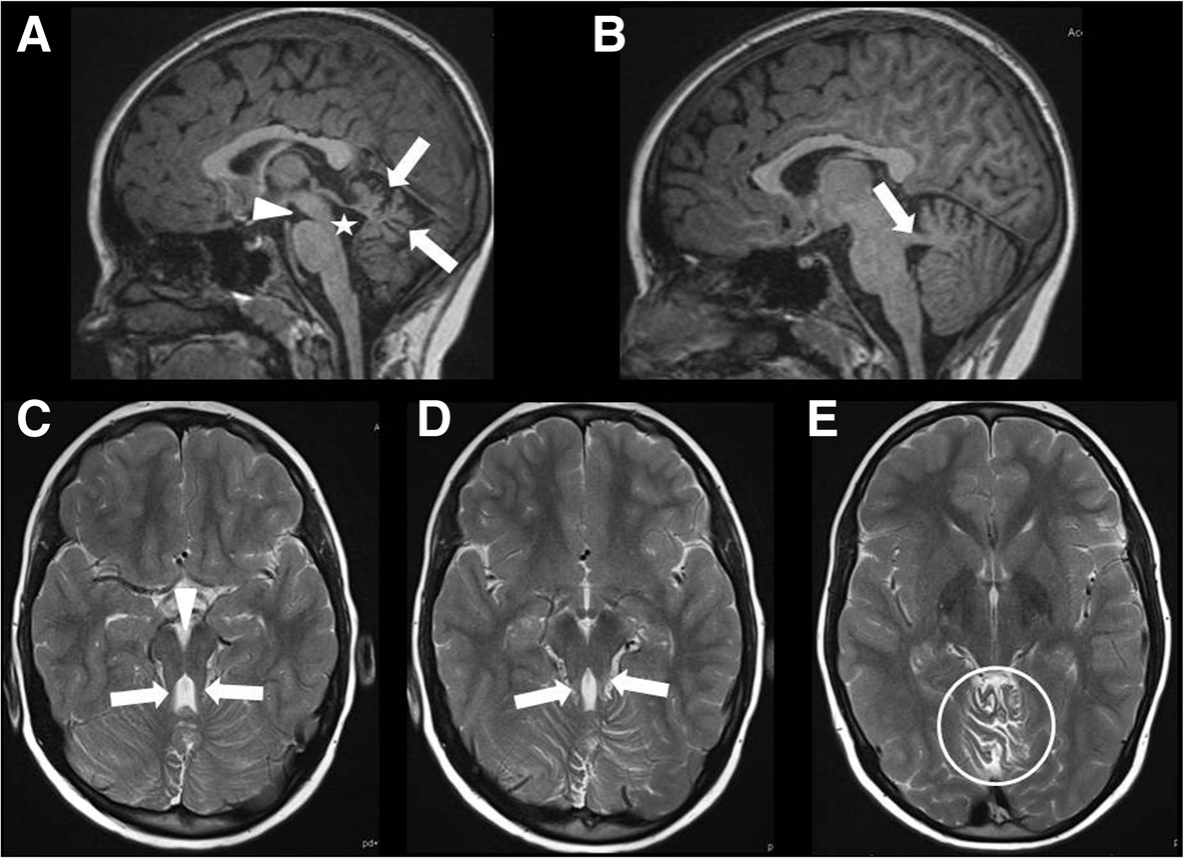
Molar tooth sign on MRI of an infant with Joubert syndrome. a, b Sagittal T1-weighted and c-d axial T2-weighted MRI of patient #1 at age 8 months. a Midsagittal slice shows vermian hypo-/dysplasia (arrows), rostral shifting of the fastigium (star), and deep interpeduncular fossa (arrowhead). b Parasagittal slice displays thickened and horizontalized superior cerebellar peduncles (SCP)(arrow). c-e Axial slices show deep interpeduncular fossa (arrowhead) and elongated SCP (arrows), resulting in “molar tooth sign”, and irregular folia of upper vermis (circle). These MRI features indicate Joubert syndrome
